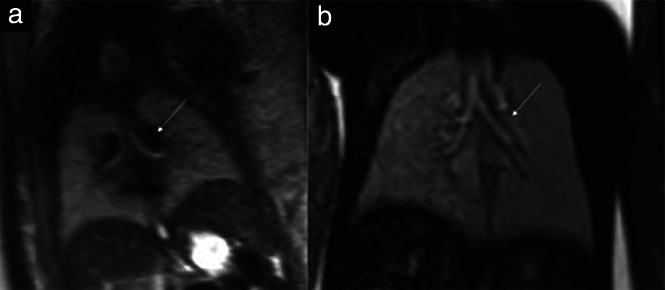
Magnetic resonance images of the pulmonary system in two fetuses with heterotaxy. (a) Fetus with right atrial isomerism at 25 + 5 weeks' gestation, presenting with abnormal branching pattern of the bronchial tree, with short bronchial arms (arrow). (b) Fetus with left atrial isomerism at 24 + 2 weeks, presenting with abnormal branching pattern of the bronchial tree, with long bronchial arms (arrow).
